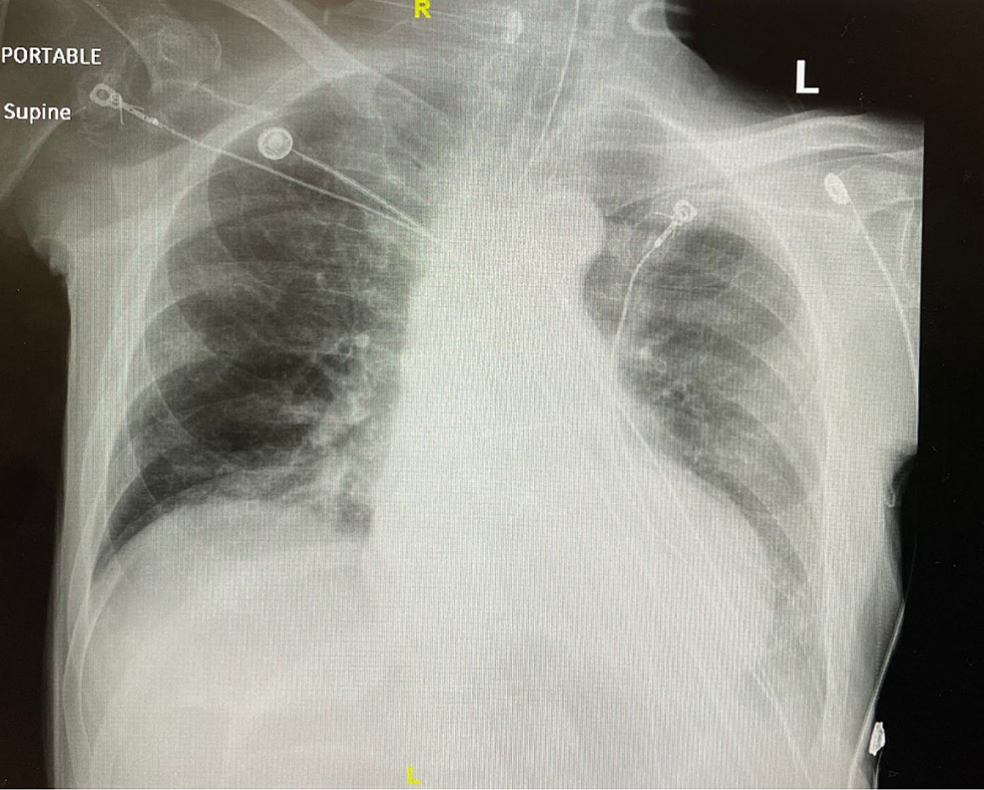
Day five postoperative CXR with resolved pneumoperitoneum. CXR, chest X-ray
